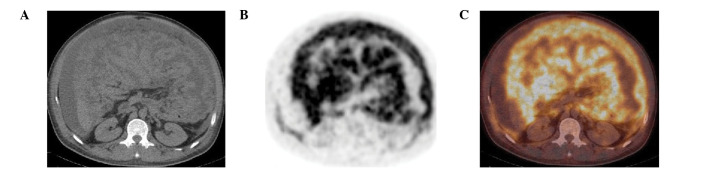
Transaxial view of (A) CT (B) PET scans and (C) fused PET/CT images showing intense FDG uptake in the peritoneum, mesenterium and greater omentum (maximal standardized uptake value, 7). CT, computer tomography; PET, positron emission tomography; FDG, fluorodeoxyglucose.
